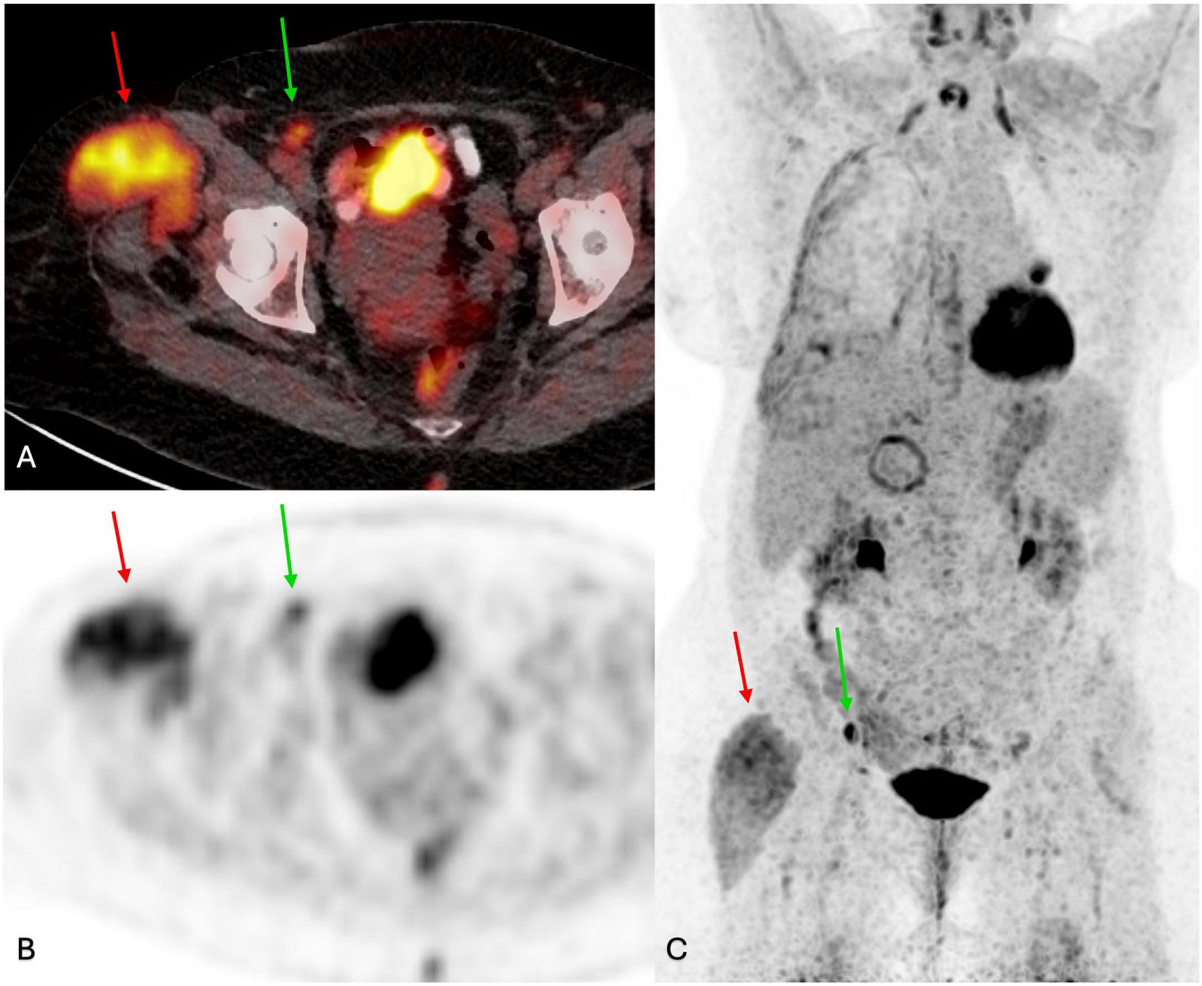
(A) Fused FDG PET/CT, (B) axial PET, and (C) PET MIP images show hypertrophied, FDG-avid, right tensor fascia lata muscle (red arrow, SUV max 5.96, background hepatic SUV max 2.86). FDG-avid inguinal lymphadenopathy is also present (green arrow, SUV max 3.58).
